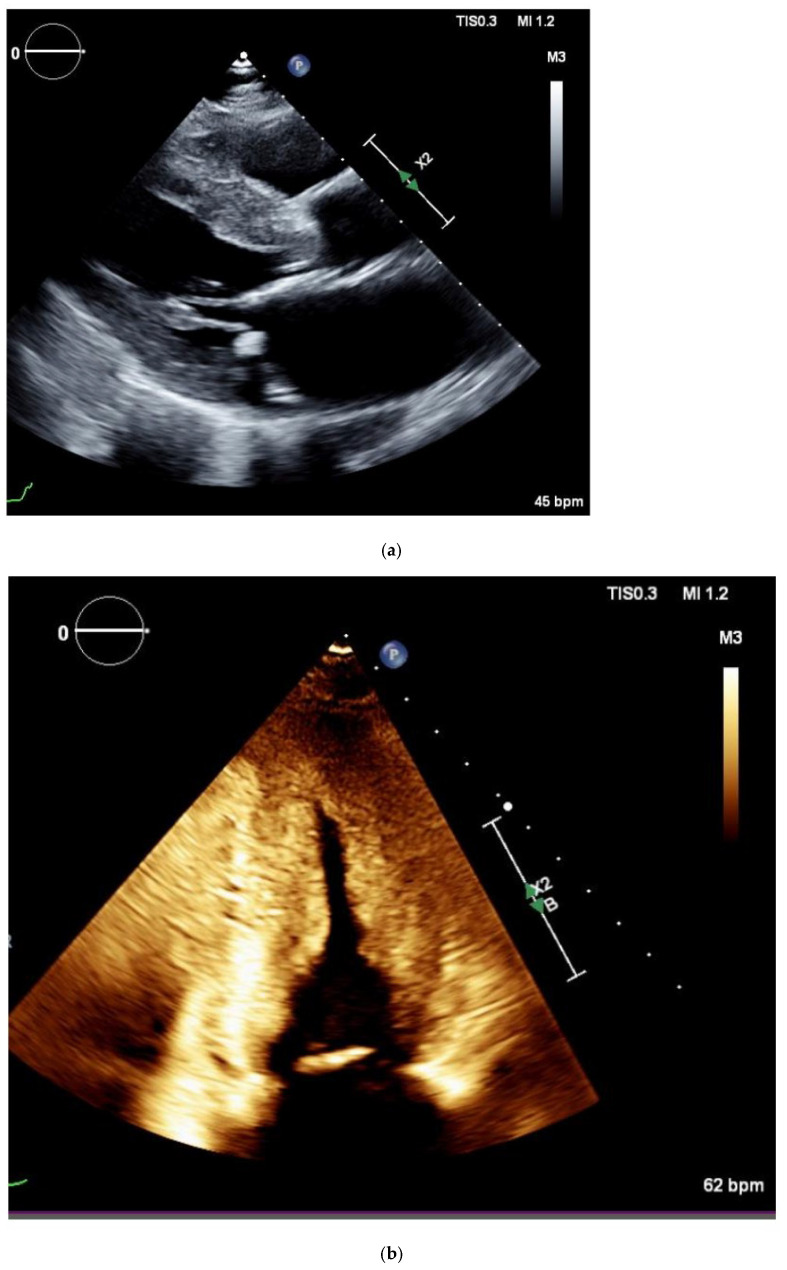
(a) Parasternal long axis echocardiogram view. Note the increased thickness of the interventricular septum. Used with permission from Sutter Sacramento Medical Center. (b) Echocardiography apical view of left ventricle. There is increased thickness of the interventricular septum and lateral left ventricle wall. Used with permission from Dr. Gurpreet Sodhi.
